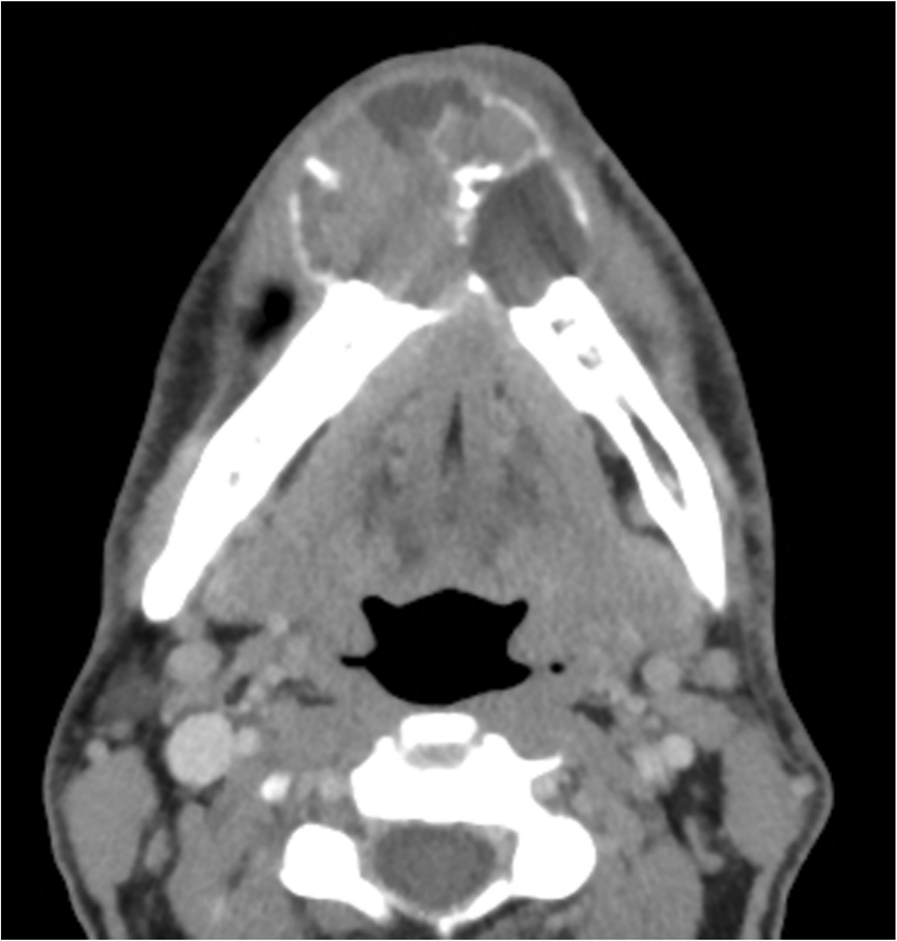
Ameloblastoma. Axial CT image shows a multilocular expansile lesion arising from the mandibular body
